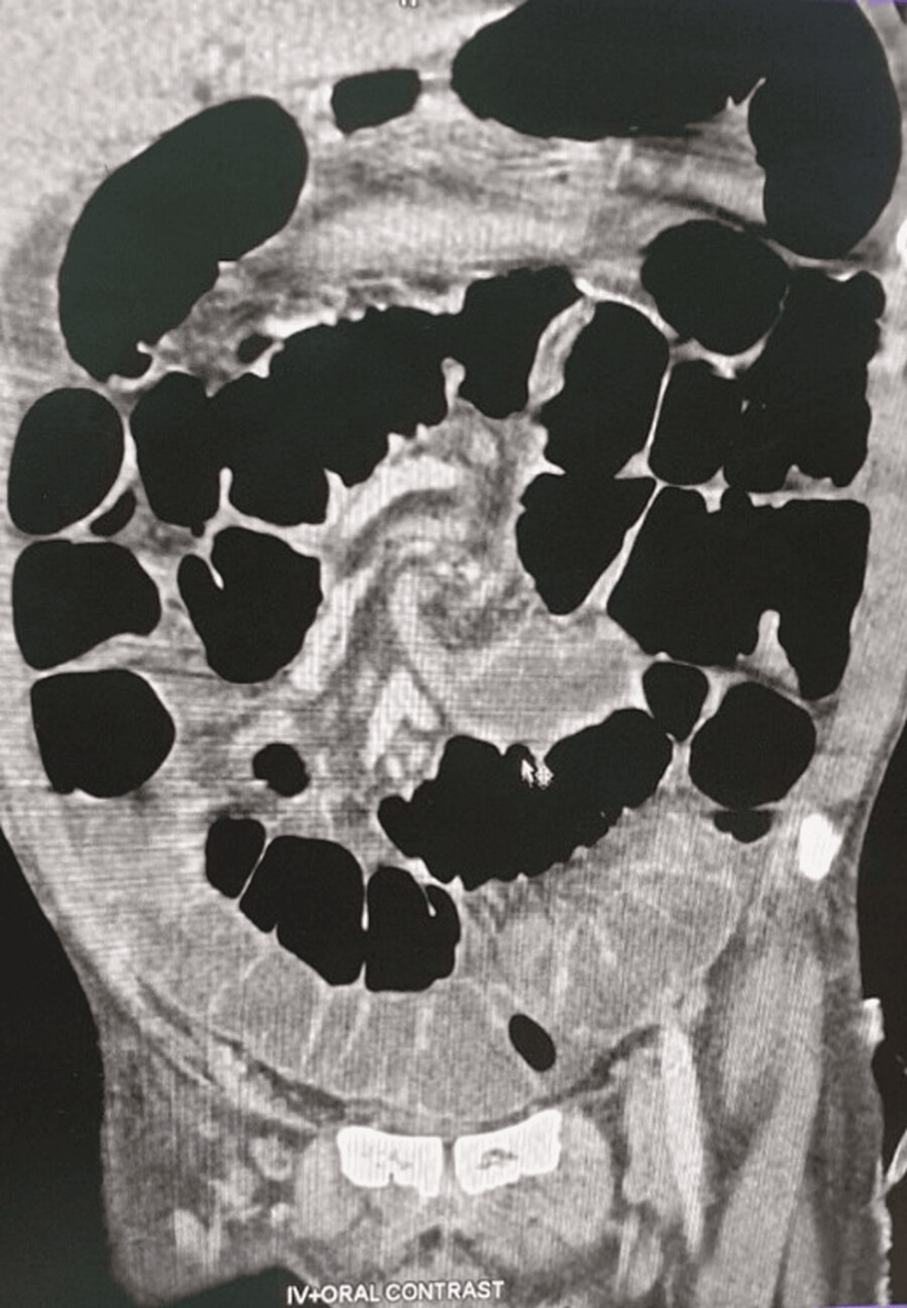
Coronal CECT view showing “whirlpool sign." Dilated ileal loop just proximal to the point of twisting and ileal segment twisting around the mesentery. CECT: Contrast-Enhanced Computed Tomography.
